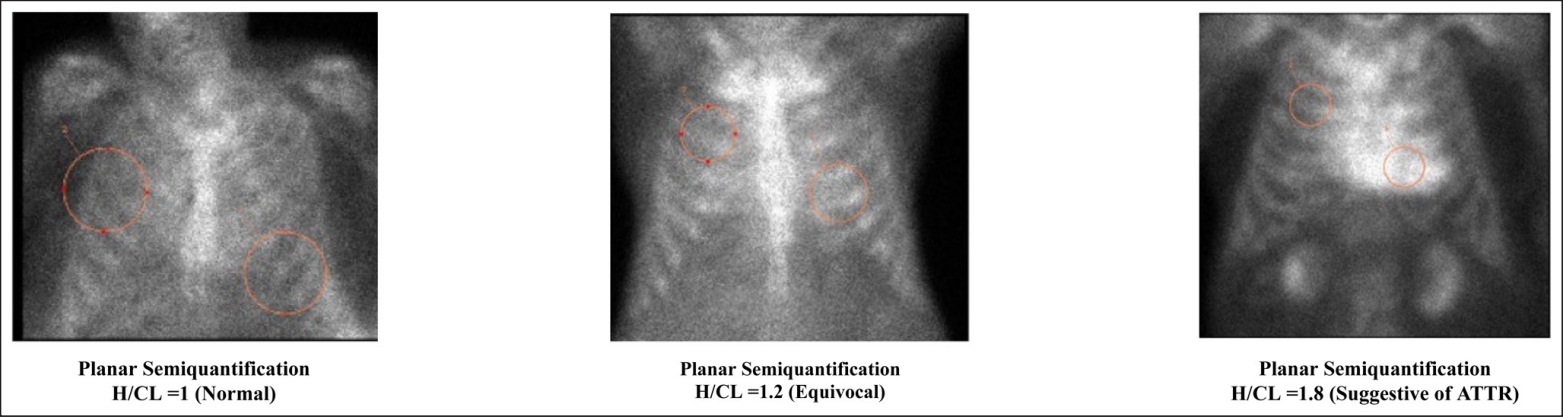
^99m^Tc-PYP cardiac scintigraphy shows anterior planar imaging using heart-to-contralateral ratio semiquantitative scoring. Reproduced with permission from Springer Nature. doi: *10.1007/s10741-021-10174-x*. H/CL: heart to contralateral lung; ATTR: transthyretin amyloidosis
